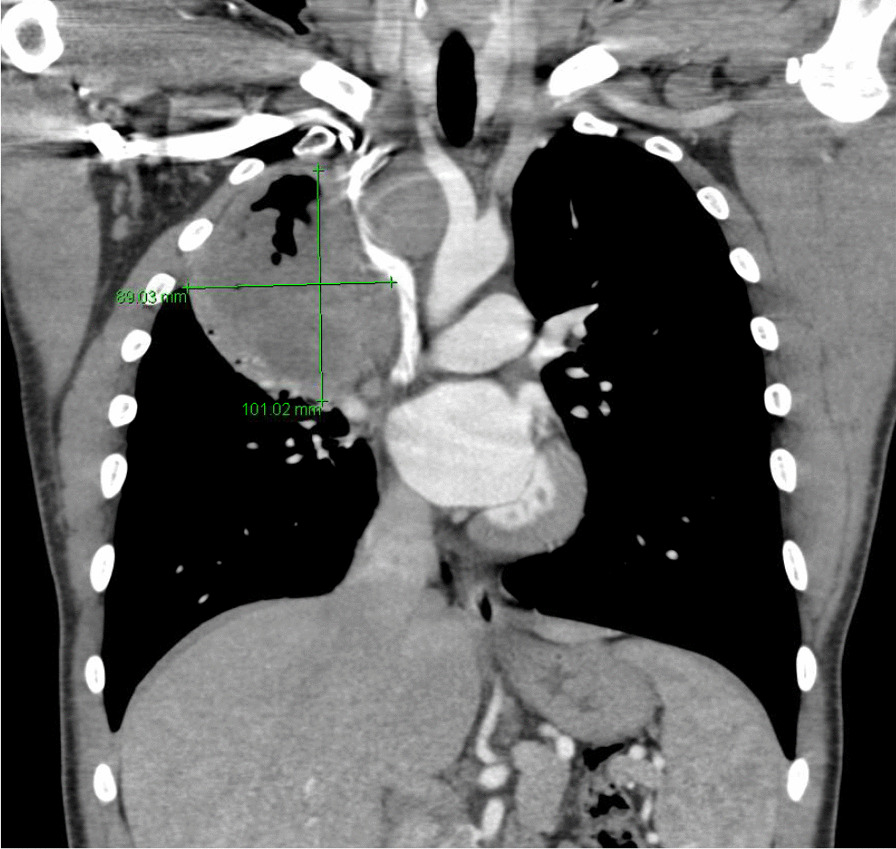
Initial CT chest with contrast (coronal image). Right upper lobe cavitary lesion with compression of the superior vena cava and azygous vein by the and lymph node conglomerate
